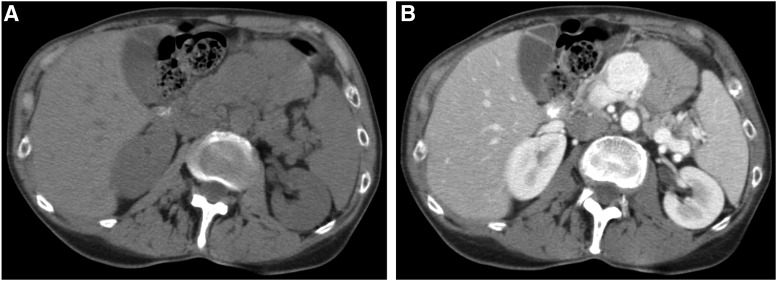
(A) Noncontrast transaxial CT demonstrates a discrete round mass in the pancreatic body. (B) Contrast-enhanced transaxial CT demonstrates avid homogeneous enhancement of the mass.
